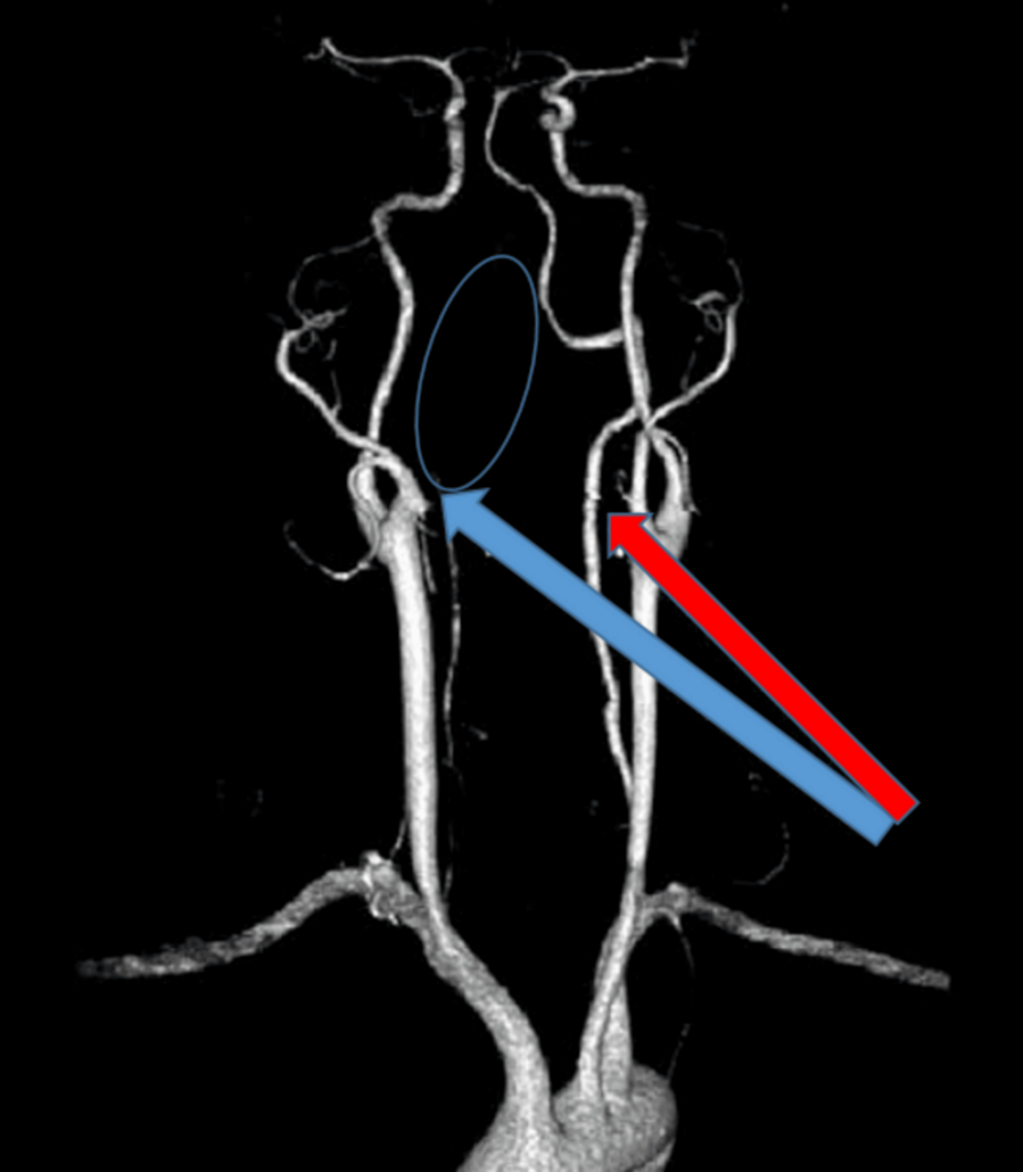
CT angiography of brain and neck vessels showing a hypoplastic right vertebral artery (blue arrow) and thrombotic occlusion of the intracranial segment of the right vertebral artery (blue circle) and the left vertebral artery (red arrow)
